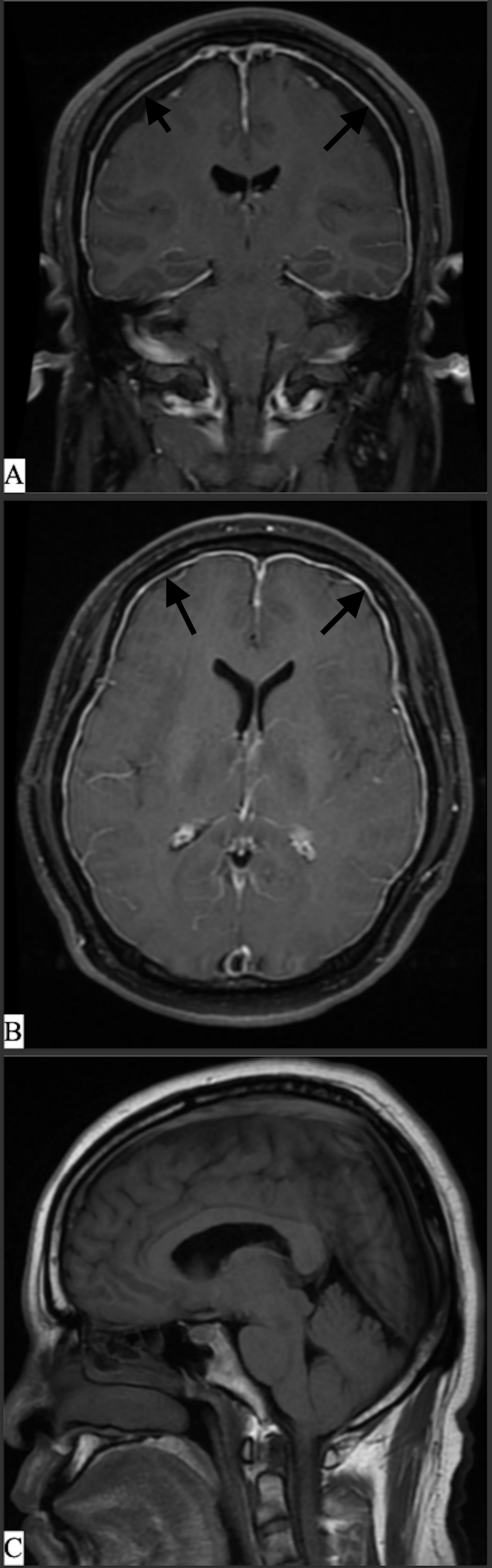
Initial MRI brain A) Initial MRI brain with gadolinium, coronal view. The patient demonstrated chronic bilateral subdural hematomas. B) MRI brain with gadolinium, axial view, demonstrating diffuse, non-nodular, pachymeningeal enhancement. C) MRI brain with gadolinium, sagittal. MRI imaging during initial workup.
